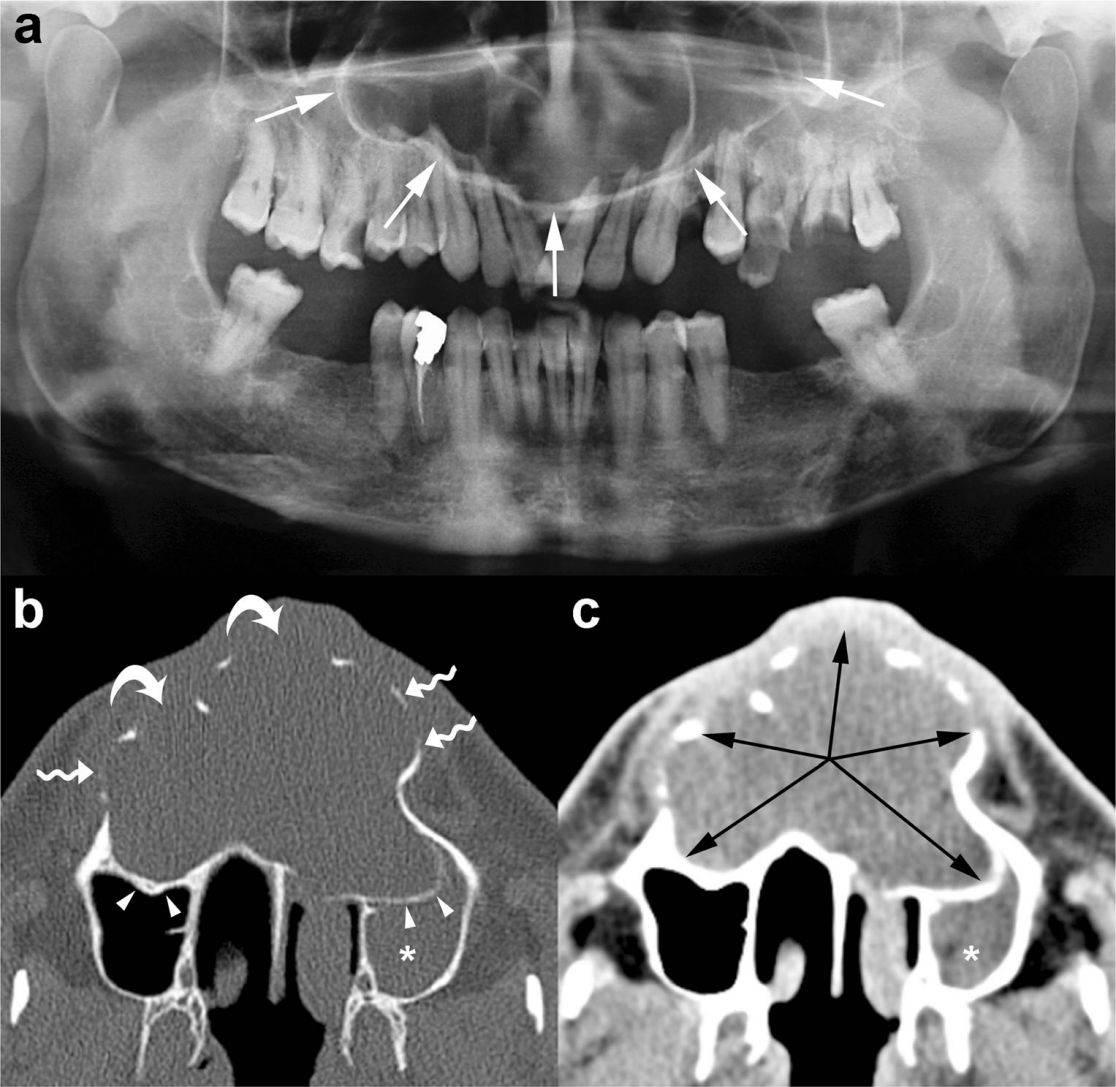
Maxillary OKC. Panoramic radiograph (a) shows a large radiolucency with a well-defined and corticated rim in the maxilla (*white arrows*). Axial multidetector computed tomography (MDCT) images with bone window (b) and soft tissue window (c) clearly demonstrate the hydraulic expansion of the maxillary alveolar bone (*black arrows*) with thinning (*wavy arrows*) and perforation (*curved arrows*) of the buccal cortex. Posterior bowing of the floor of the maxillary sinuses (*arrowheads*) and inflammatory material within the left maxillary sinus (*asterisks*) are also shown
